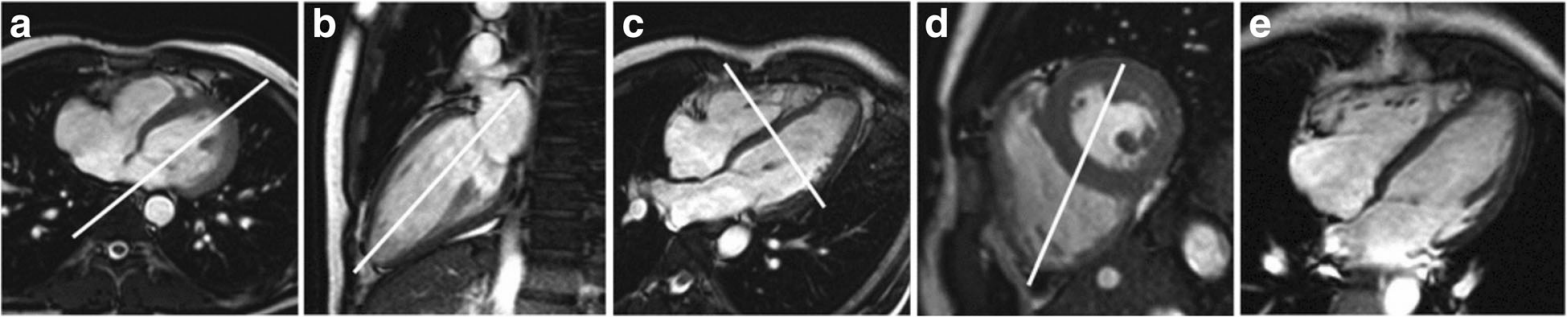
Cardiac MRI planes [7]. a Axial plane. b Vertical long-axis plane. c Horizontal long-axis plane. d Short-axis plane. e Four-chamber plane
